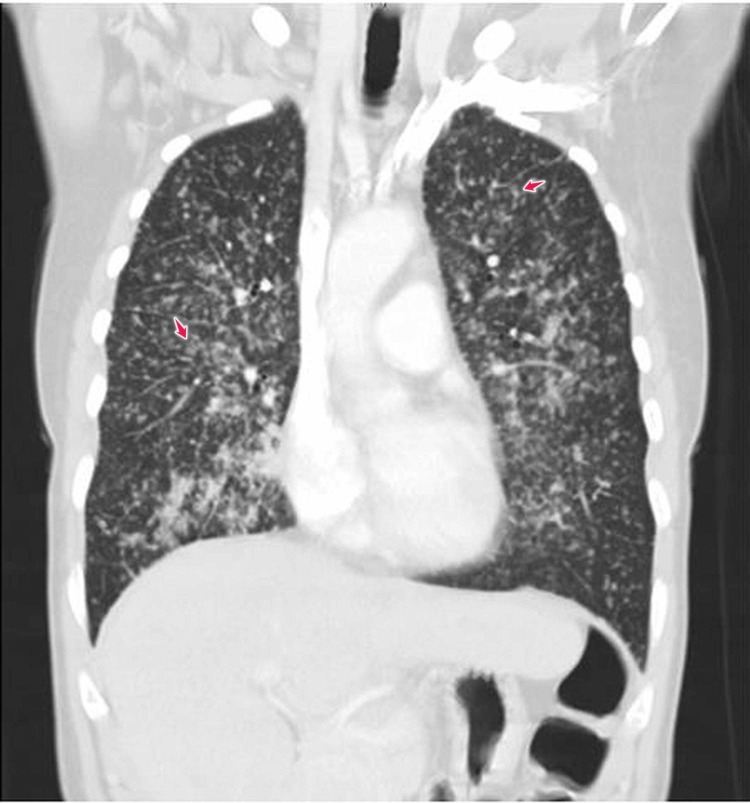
CT scan chest Chest computerized tomography (CT) scan with multiple bilateral miliary nodular infiltrates (arrows)
